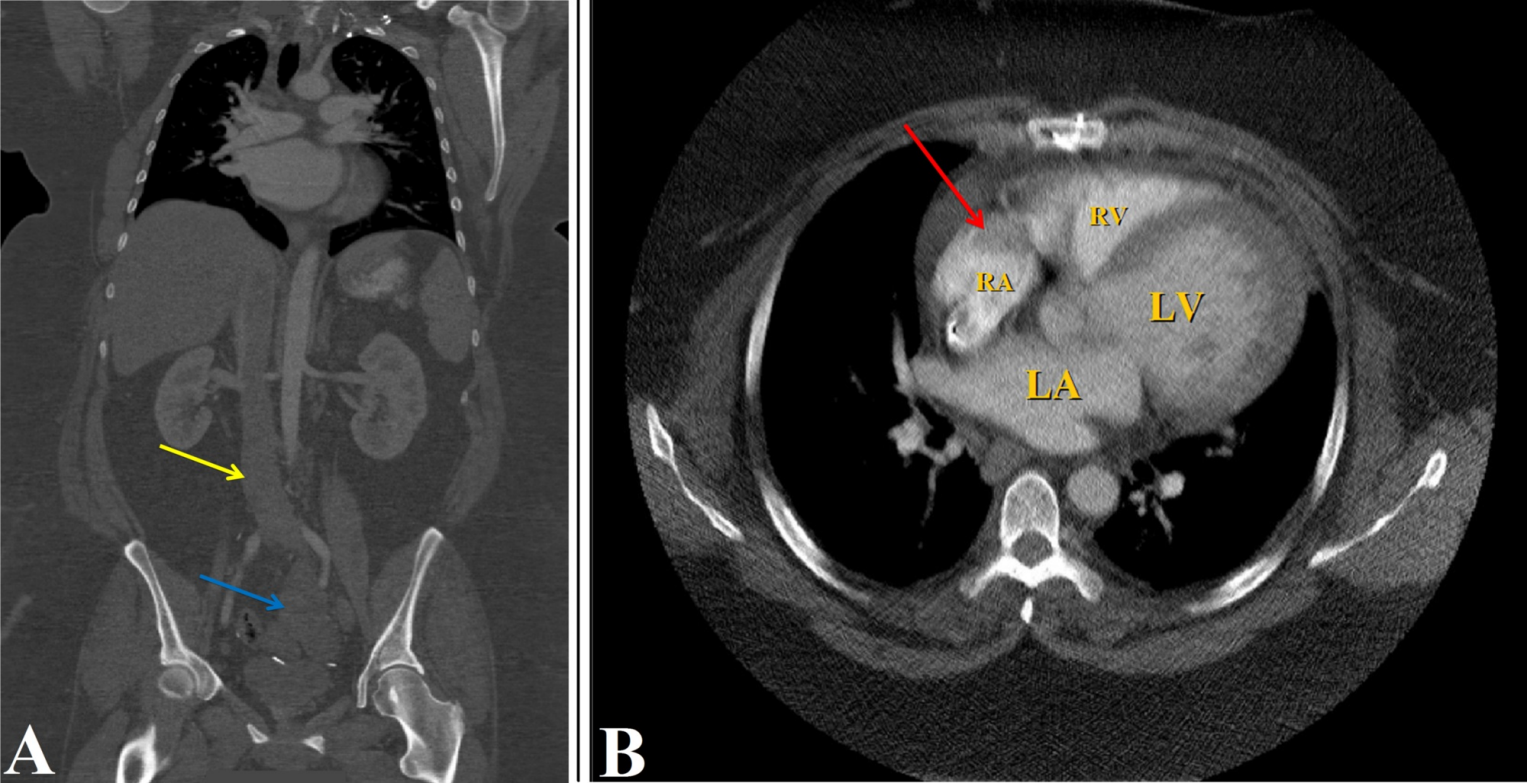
Contrast-enhanced CT abdomen and pelvis showing: (A) nonocclusive IVC thrombus extending to the intra-hepatic IVC into the iliac veins (yellow arrow), and left adnexal mass (blue arrow); (B) thrombus in the right atrium (red arrow). IVC, inferior vena cava; LA, left atrium; LV, left ventricle; RA, right atrium; RV, right ventricle
